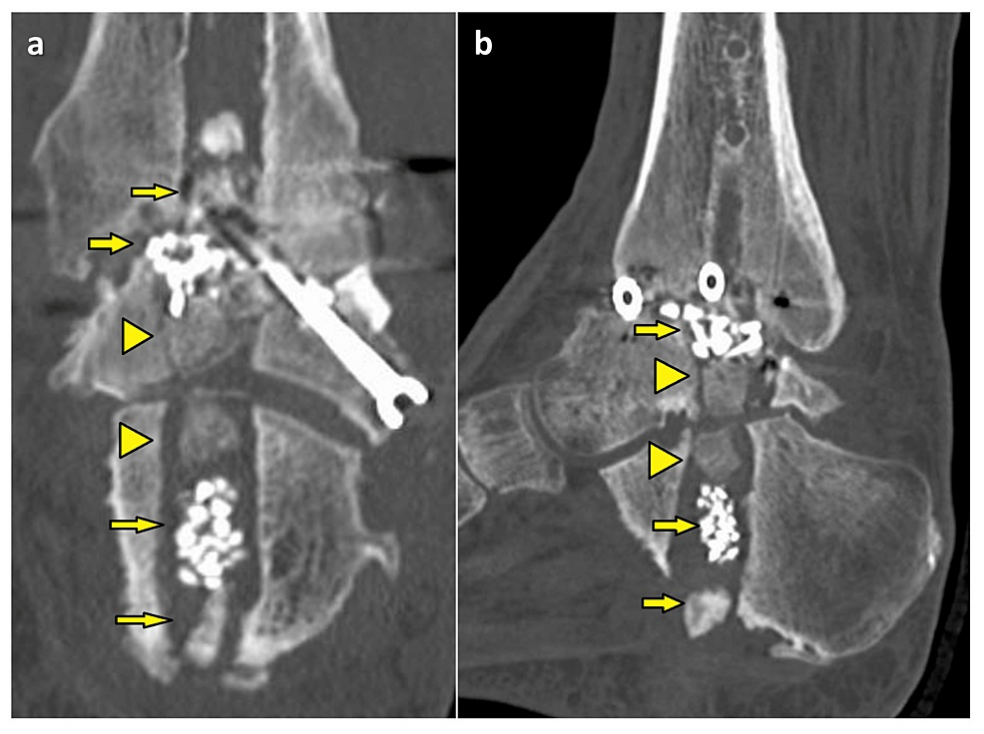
CT at one week after surgery (a) coronal and (b) sagittal images Autologous cancellous bone and artificial bone were filled in the ankle joint and intramedullary nail canal in the calcaneus (Arrows). Two osteochondral plugs (Arrowheads) were placed to reconstruct the subtalar joint. CT, computed tomography
